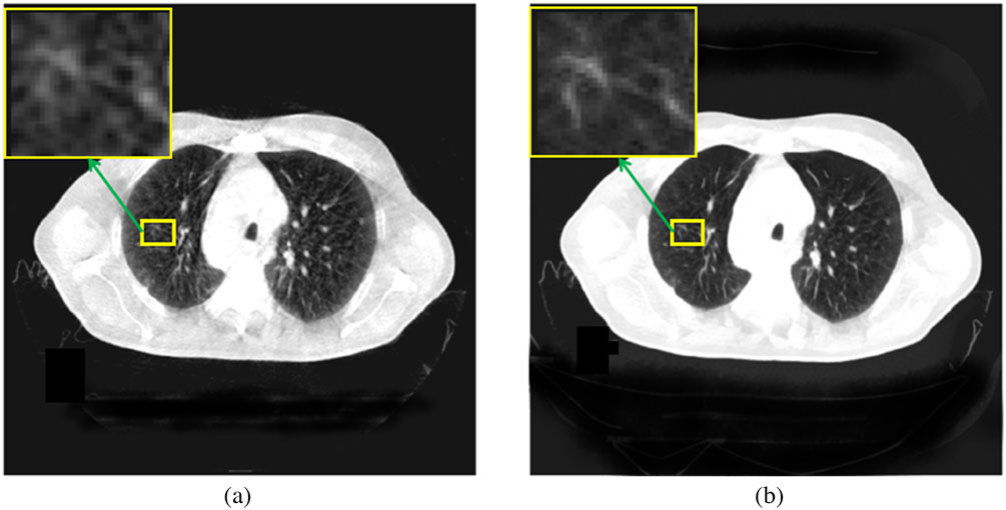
Vacuole sign tumor. Display window [−100,1063]. (a) The original CBCT image; (b) the correction result of our proposed method.
